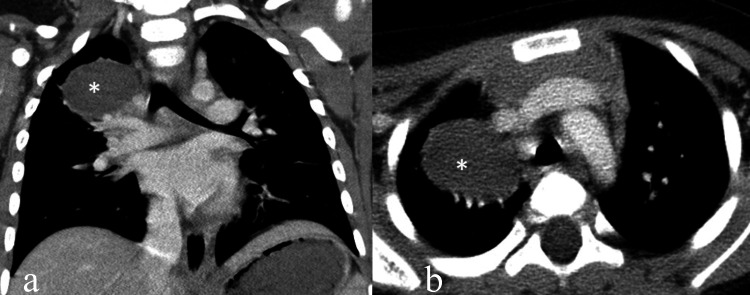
Follow-up CT (three months) Follow-up CT images in coronal (a) and axial (b) plane reveal cyst lesion (star) with no evidences of infection.
